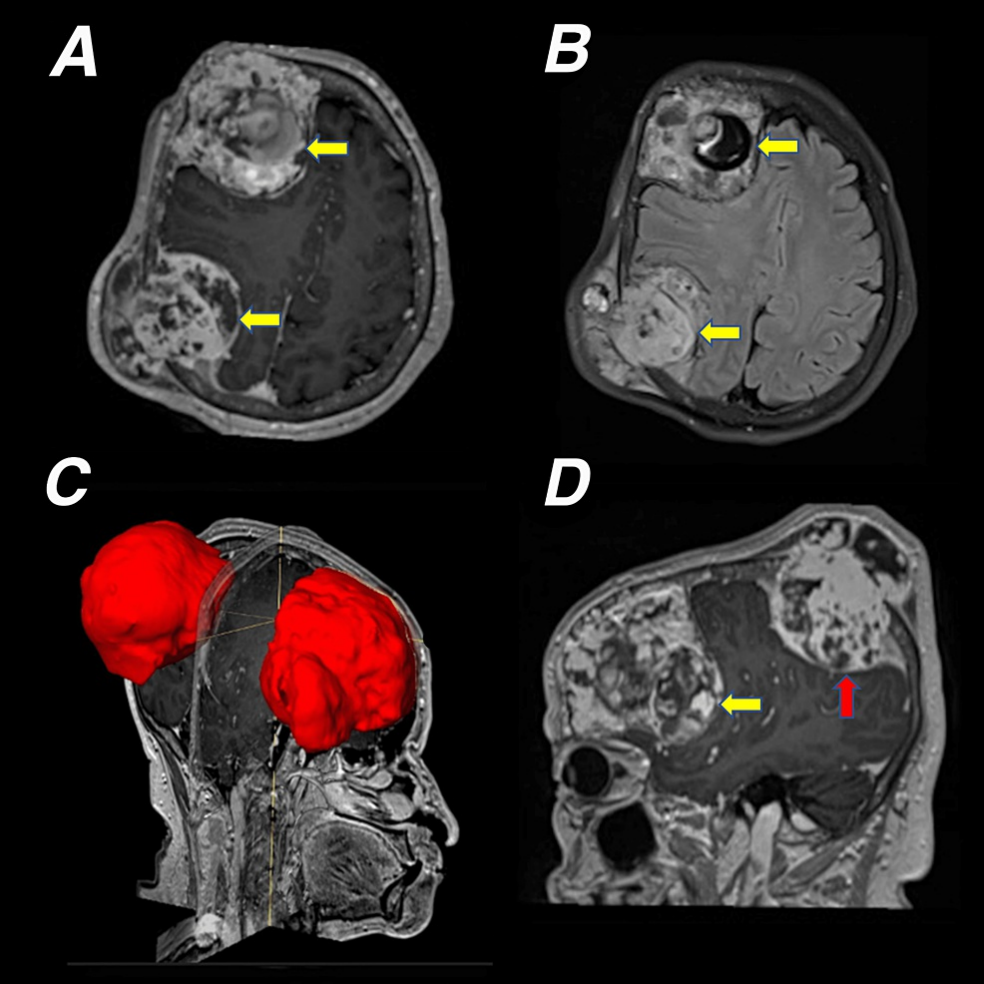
Brain magnetic resonance imaging. (A) Axial section in T1 sequence with right hemisphere lesions with bone and soft tissue invasion and intracranial and extracranial components; enhancement of the solid portions after intravenous contrast medium (yellow arrows). (B) An axial section in FLAIR sequence shows two multilobed and heterogeneous lesions with a hyperintense signal compatible with necrosis areas (yellow arrows). (C) Right sagittal section in 3D reconstruction sequence of both lesions and their relationship with the brain and skull. (D) The left sagittal section in T1 sequence with intravenous contrast medium shows the largest lesion in the frontal and temporal lobes with secondary compression of the lateral ventricle. Surrounding edema and displacement of midline structures up to 2 cm to the left (yellow arrow). The parietal lesion is compressing the parenchyma and displacing the basal nuclei and the mesencephalic peduncle (red arrow). FLAIR: fluid-attenuated inversion recovery; 3D: three-dimensional.
